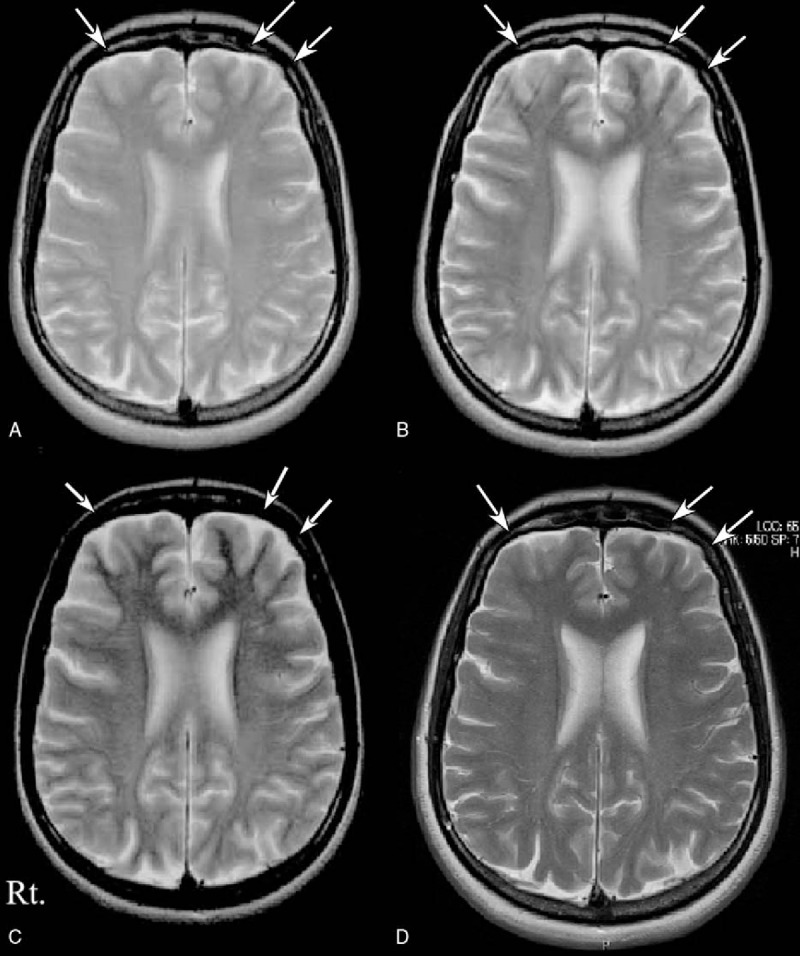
Serial MRI studies in Patient 1 (upper panels). A second brain MRI obtained 16 days after admission (panel B) showed the development of diffuse brain atrophy as compared with first brain MRI obtained on admission (panel A). Brain MRI obtained 6 months after admission showed the further progression of diffuse brain atrophy (panel C). Fourteen years after admission, the diffuse brain atrophy had partially improved (panel D).
